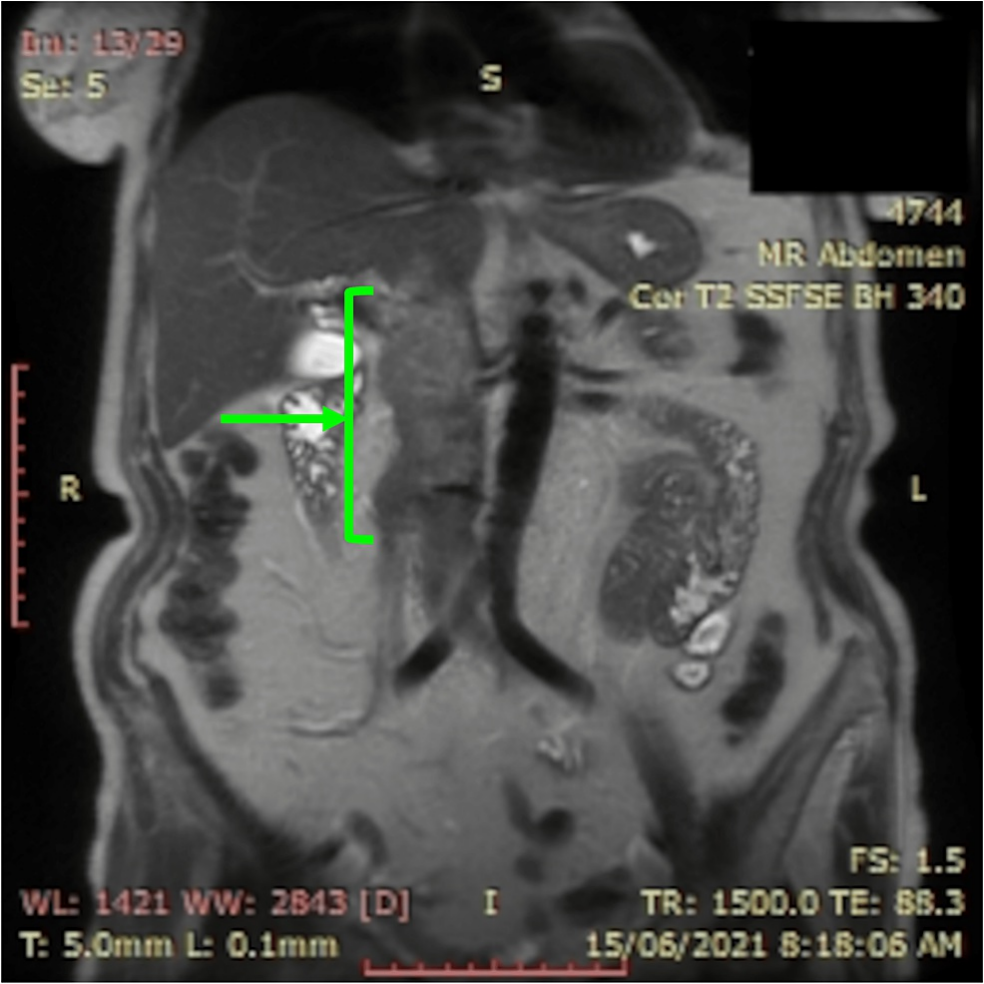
MRI of the abdomen displaying a 10 cm filling defect in the IVC, with the inferior margin of ≈5.8 cm proximal to the aortic bifurcation and its superior margin in the intrahepatic portion of the IVC. MRI, magnetic resonance imaging; IVC, inferior vena cava
